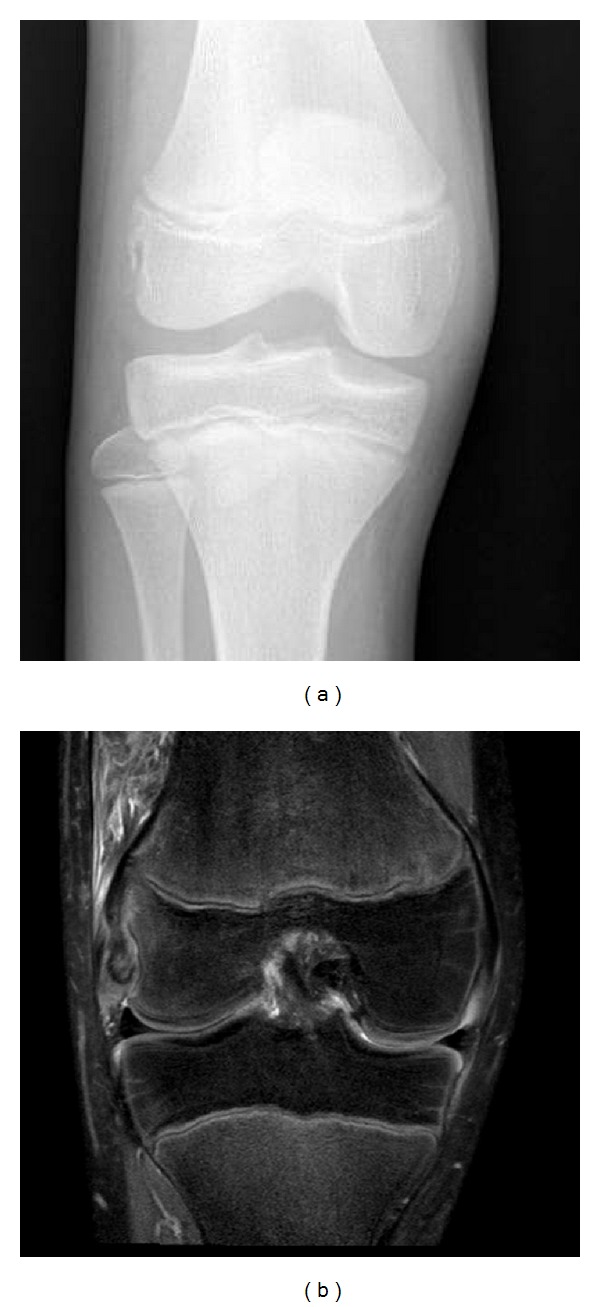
(a) Case 1 injury AP knee showing lateral distal femoral avulsion fracture. (b) Case 1 T2 MRI showing popliteus tendon avulsion.
